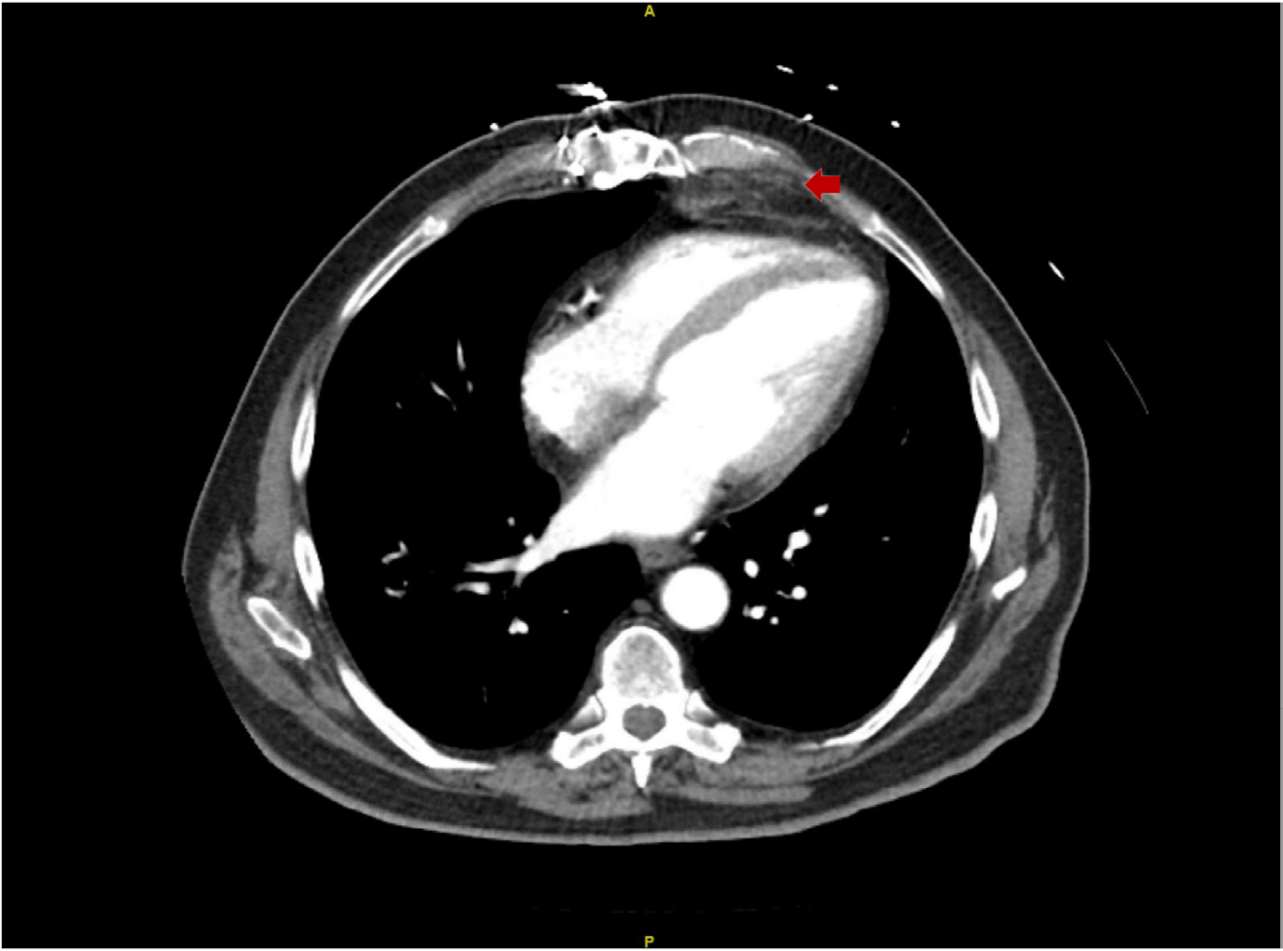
CT of chest showing anterior thoracic mass (red arrow).
